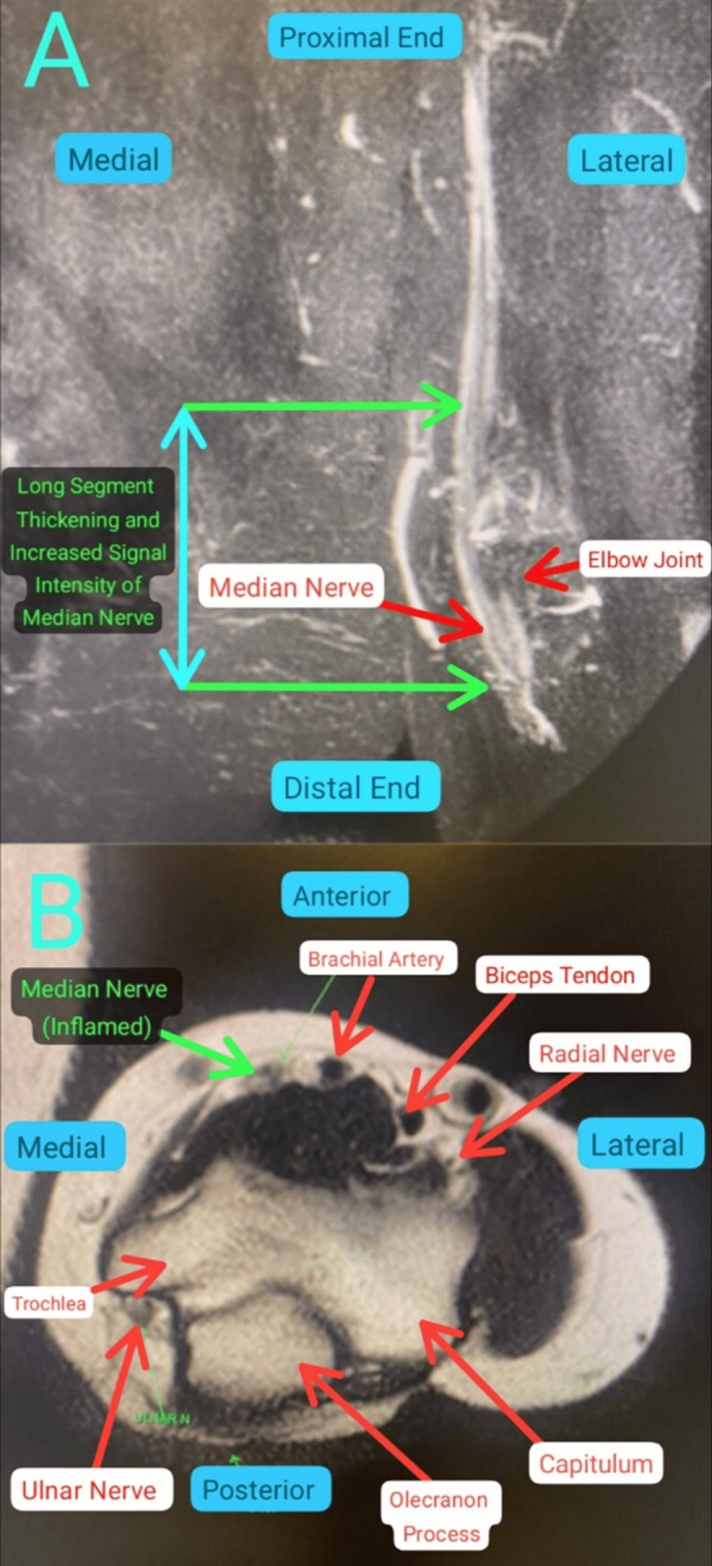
T2-weighted Fat Sat MRI of left elbow joint. (A) Coronal view; (B) axial view show long segment thickening and increased signal intensity of median nerve above and below the elbow joint (Green Arrows) upto the insertion of biceps tendon. About 11 cm of median nerve is inflamed from supracondylar region of humerus to the point where it enters between the two heads of pronator teres. Features are suggestive of median nerve compression neuropathy. Scale bar is 1:1.
